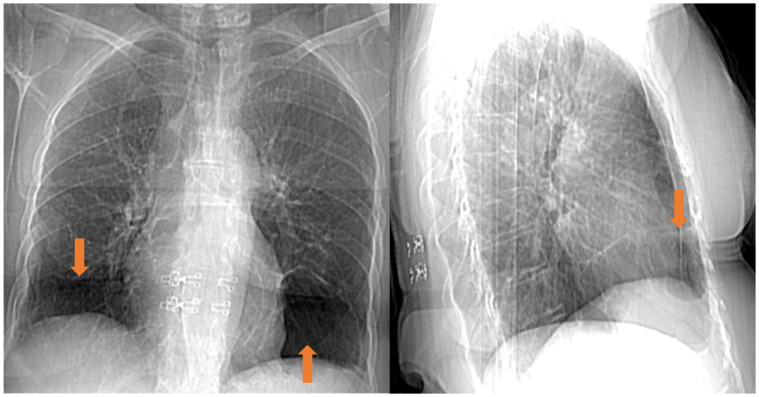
Chest radiograph of a patient with BHD syndrome showing thin-walled cysts (orange arrows), predominantly located in the lower lobes of the lungs.
